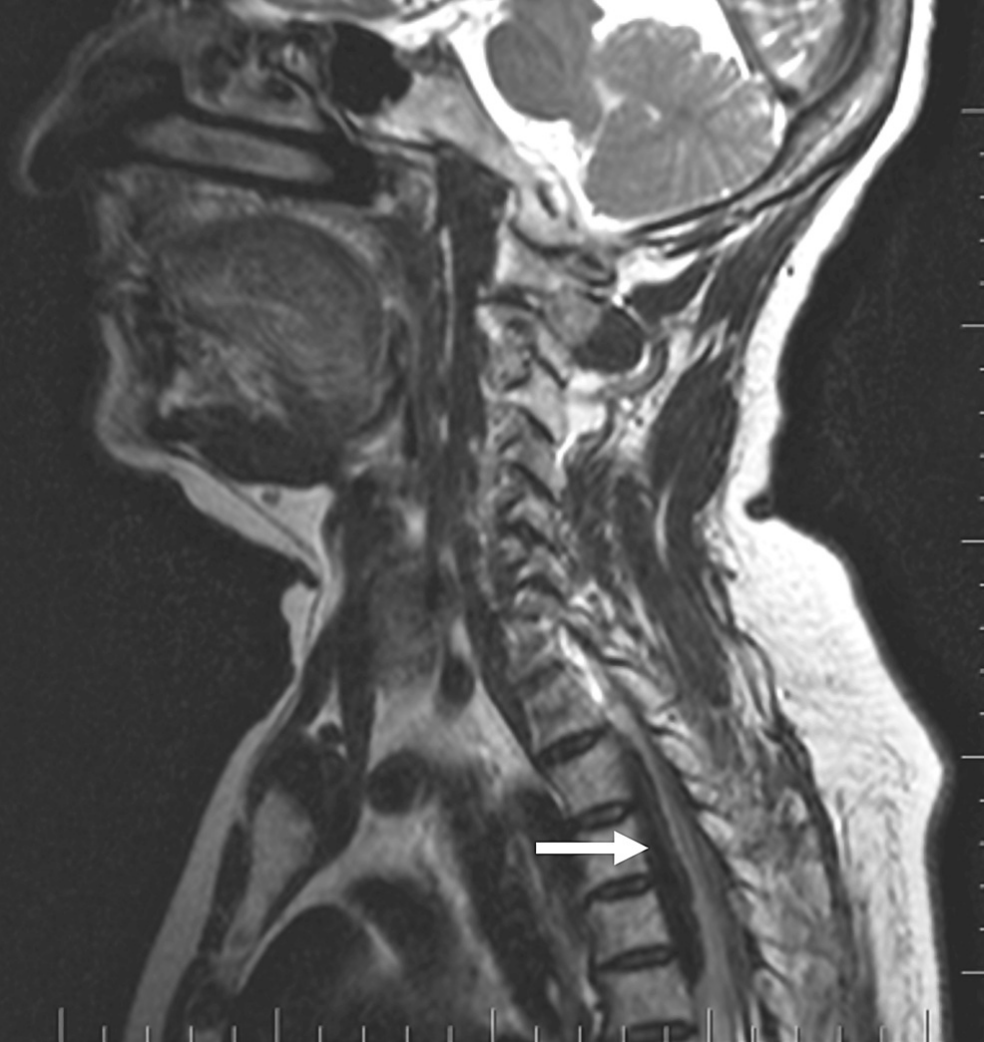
Preoperative MRI (T2 sagittal) showing a well-defined extradural and ventrodorsal lesion causing severe impingement of the spinal cord (arrow).
